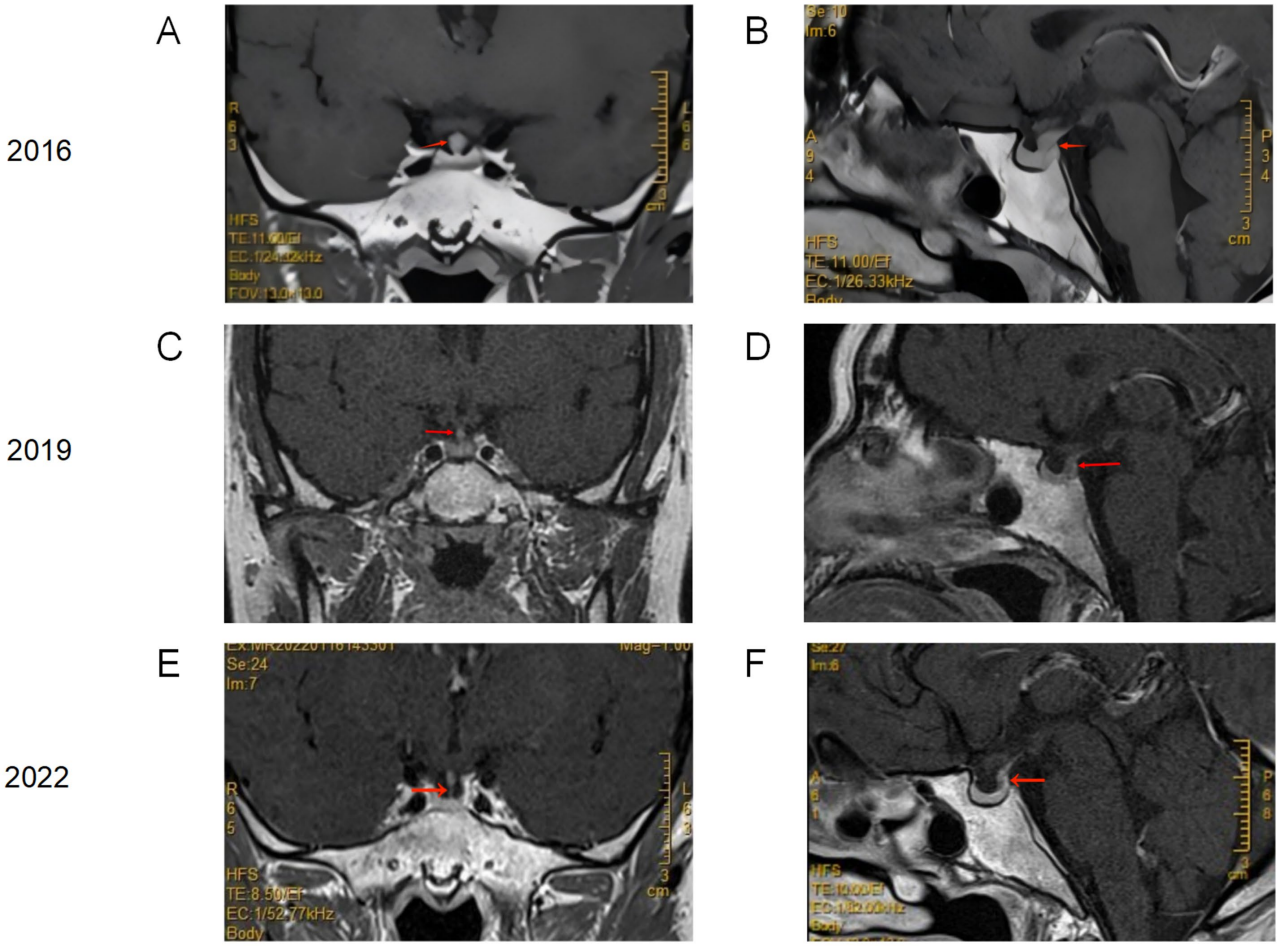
Longitudinal cranial MRI Follow-up of the LCH Patient. (A,B) Post-radiotherapy MRI of the pituitary gland (2016) demonstrating a slight reduction in pituitary volume with diffuse pituitary stalk thickening, uniform enhancement, and indistinct posterior pituitary visualization. (C,D) Underwent a follow-up cranial MRI at our hospital in 2019. Comparative analysis with imaging from February 16, 2016, demonstrates a subtle volumetric reduction of the pituitary gland. The infundibulum continues to exhibit nodular thickening with a homogeneous enhancement pattern, though notably diminished in diameter compared to the 2016 examination. (E,F) After being diagnosed with LCH and chemotherapy in 2022. Comparative analysis with imaging from December 17, 2019, reveals preserved adenohypophyseal dimensions. The pituitary infundibulum demonstrates physiological calibre with uniform morphology, homogeneous contrast enhancement, and absence of space-occupying lesions.
